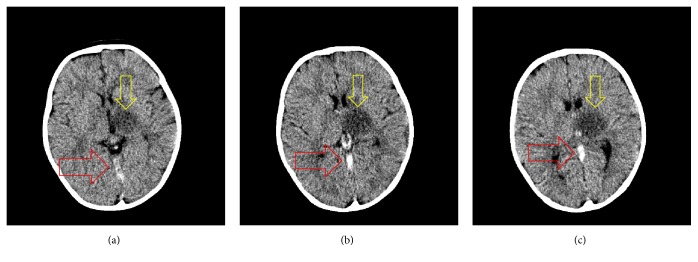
Axial noncontrast CT at the level of basal ganglia. These scans demonstrate dense clot sign which show a dark area (yellow arrows) where the infarct of the left thalamus is located due to thrombosis of internal cerebral veins and straight sinus (red arrows).
